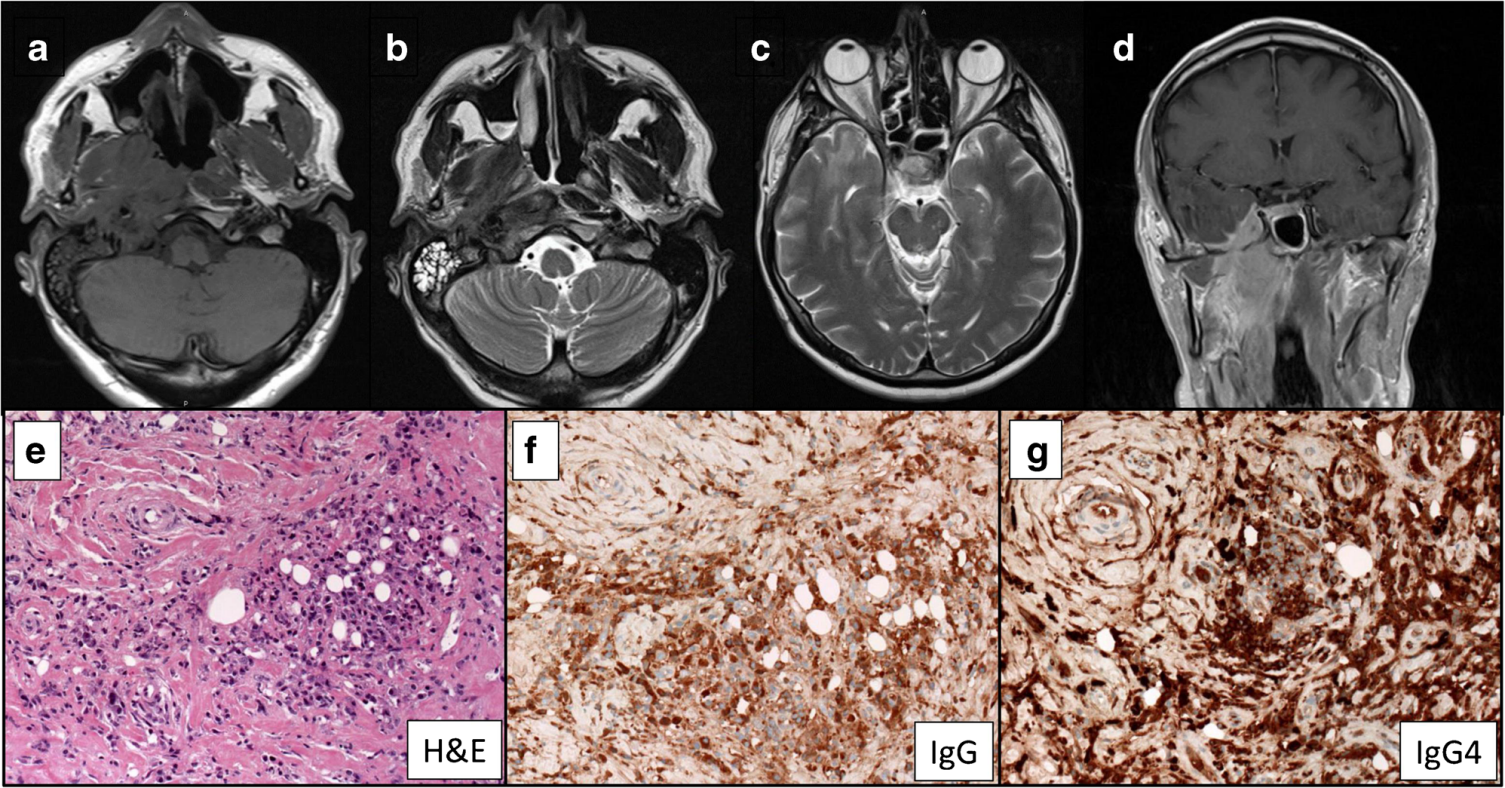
MR imaging (a, b) demonstrated an enhancing soft tissue mass involving the right posterior nasopharynx with infiltration laterally and posteriorly into the right prevertebral strap muscles and through the pharyngobasilar fascia to involve the medial and lateral pterygoids. The right carotid and internal carotid artery was ensheathed and abnormal tissue was seen in the right carotid canal and jugular foramen. The right cavernous sinus was involved via perineural spread through the foramen ovale. Basal pachy- and leptomeningitis were noted along the floor of the right middle cranial fossa with high signal in the overlying temporal lobe (c). Progressive changes were noted in the pterygomaxillary fissure, the muscles of the masticator compartment and throughout the temporalis muscle on the right at the time of representation (d). e–g A diffuse plasma cell-rich, chronic inflammatory cell infiltrate with prominent stromal fibrosis/hyalinization, fat necrosis and focal granulation tissue was evident on biopsy of the anterior temporalis and posterior maxilla (e). Immunocytochemistry demonstrated numerous IgG- and IgG4-positive plasma cells (f, g). Reproduced with permission from Rice et al. [95].
